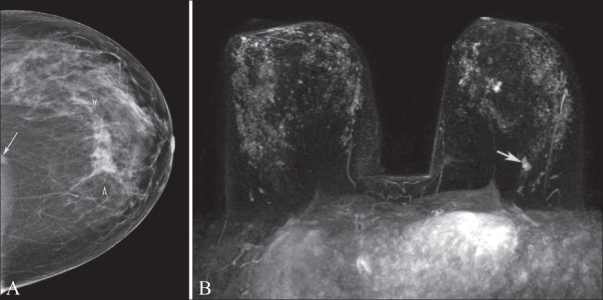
Mammogram, craniocaudal projection (A) and maximum-intensity projection (MIP) DCE-MRI (B). This was a 48-year-old woman noted to have a density (arrow) in the craniocaudal projection only and architectural distortion (arrowheads) in the subareolar location in both standard mammographic views. The anterior lesion was biopsied and proved to be invasive mammary carcinoma with ductal and lobular features. The density could not be identified on multiple additional diagnostic views. DCE-MRI was recommended to further evaluate the initial mammographic finding. The lesion (arrow) was identified by MRI and located at the 6 o'clock position, far posterior to the chest wall. This lesion was also biopsied and proven to be a second area of invasive mammary carcinoma
